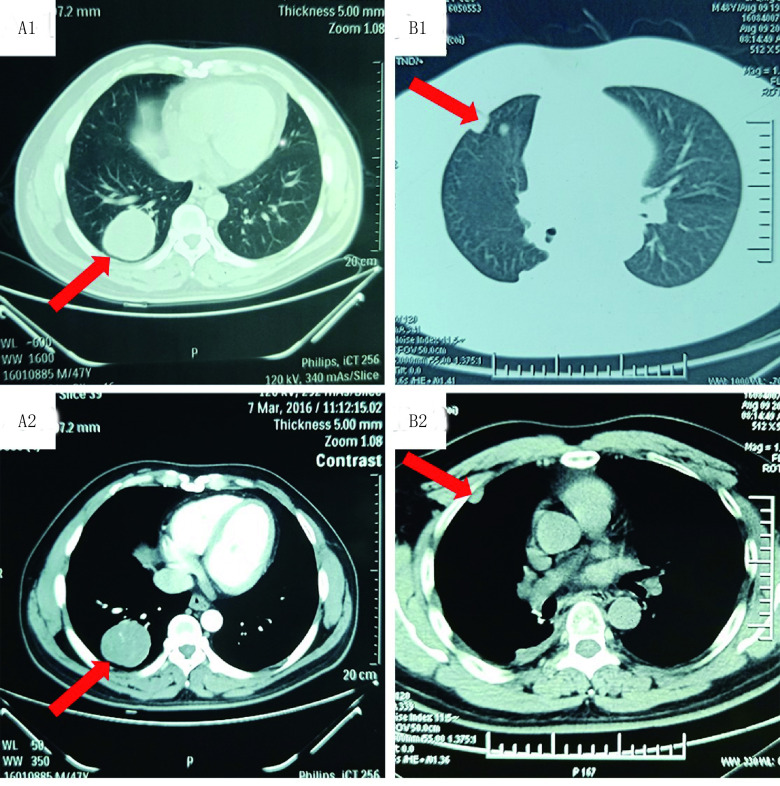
术前及术后进展的CT扫描图像：2016年3月CT扫描显示右肺占位（A1、A2）；术后定期复查，2016年8月病情进展（B1、B2）。 Chest CT scans before and after taking operation. March 2016 CT scans showed that a mass in the right lung (A1, A2); August 2016 CT images shows progression disease (B1, B2). CT: computed tomography.
